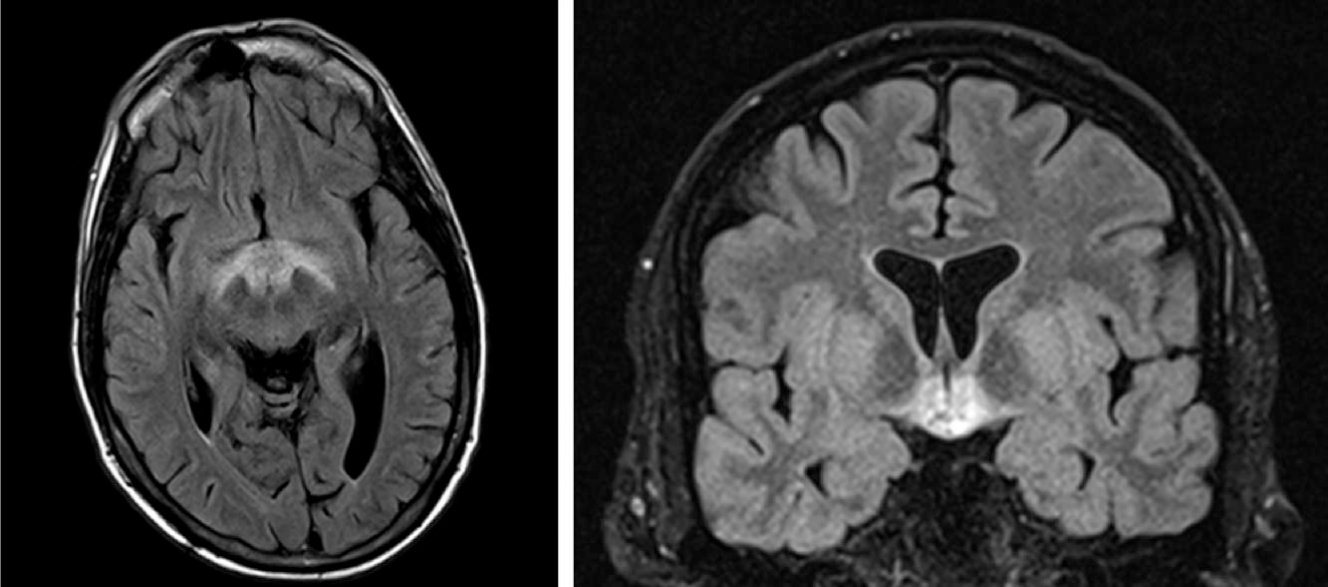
Magnetic resonance imaging of the brain 4 days into admission and 3 days after fluid restriction.
